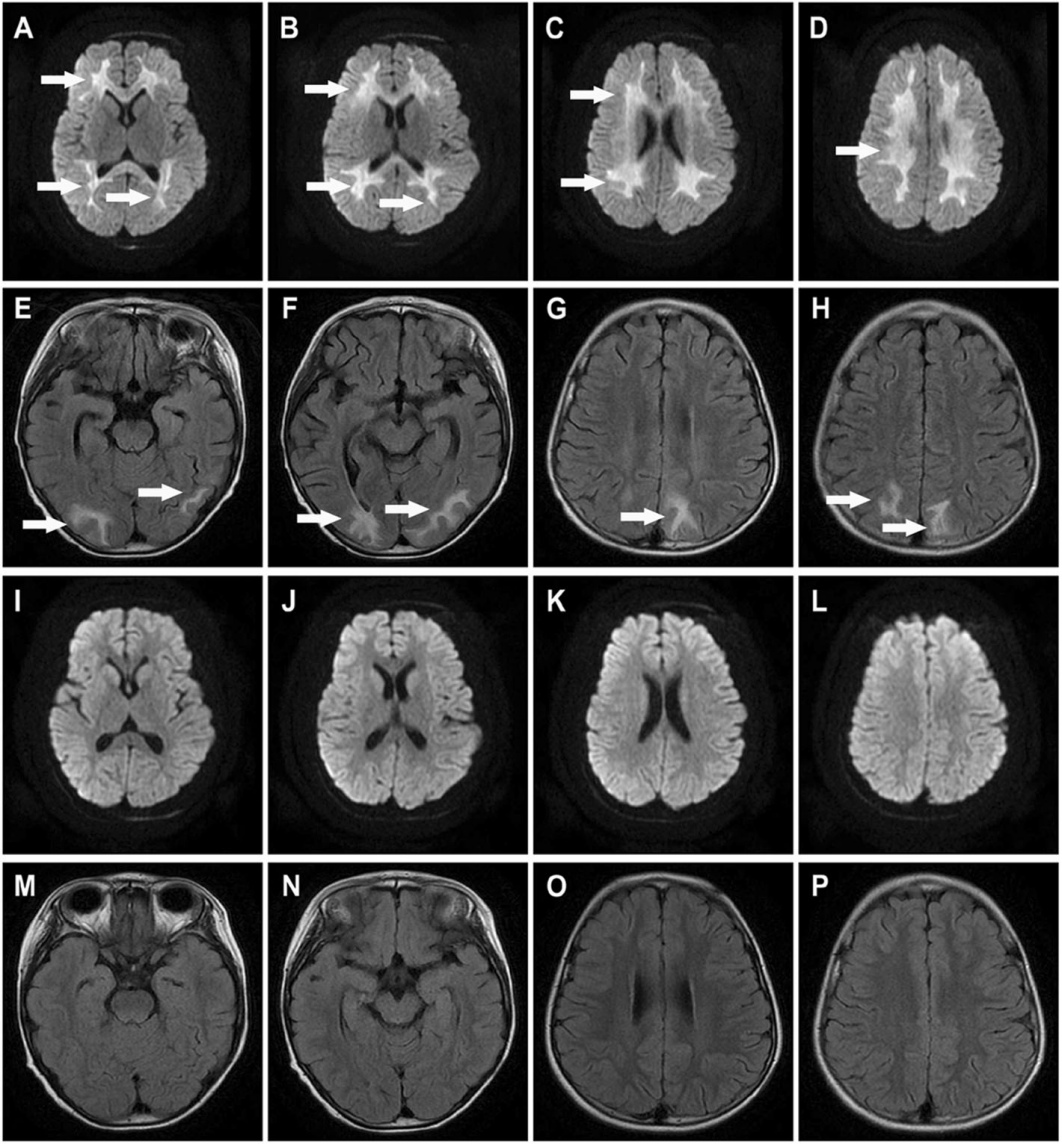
Brain magnetic resonance imaging (MRI) (a-d) DWI showed symmetric areas of high signal intensity in the periventricular white matter involving the centrum semiovale and corona radiate on day 1 (white arrow). (e-h) T2/FLAIR images showed diffuse high signal intensity in the white matter of occipital and parietal regions on day 17(white arrow). (i-p) DWI and T2/FLAIR images showed brain lesions had completely disappeared in the white matter on day 55
